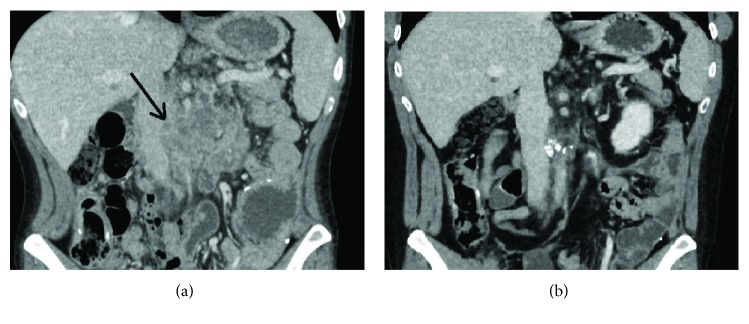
CT contrast imaging before (a) (October 8, 2015) and after (b) (February 1, 2019) administration of pembrolizumab. The black arrow in panel (a) indicates a large retroperitoneal lymph node conglomerate which compressed the inferior vena cava, encased the aorta, and invaded the left renal vein. The lymph node conglomerate has since been reduced to small dense calcifications as seen in panel (b).
